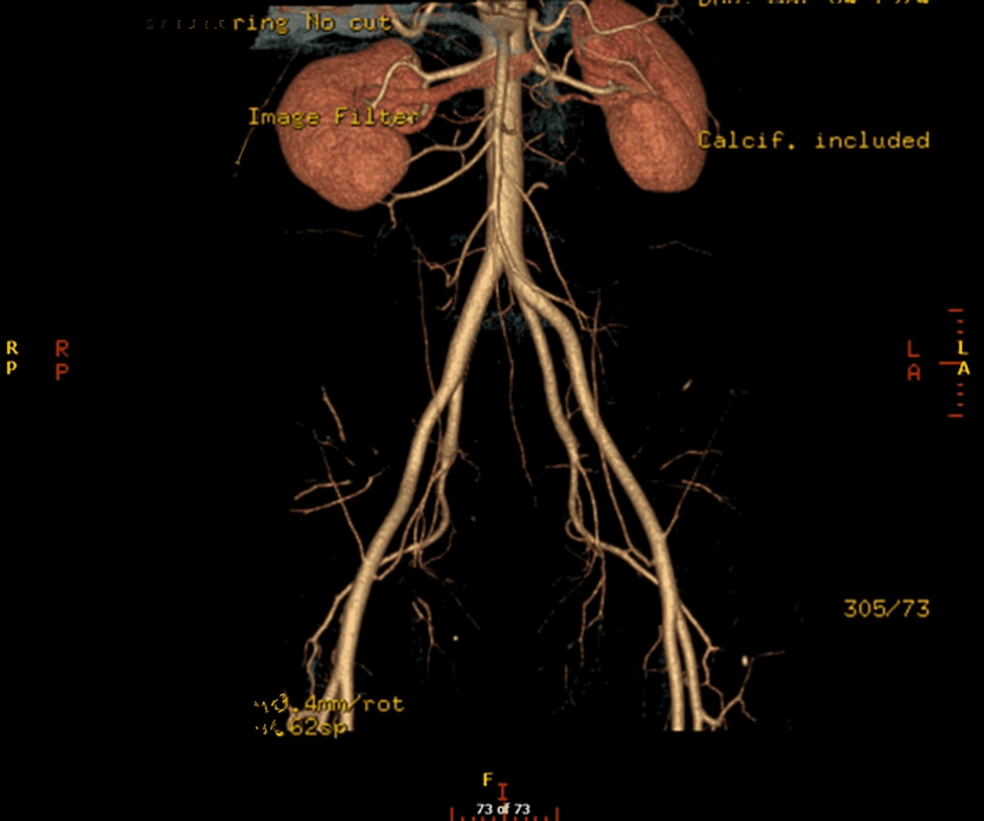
Three-dimensional reconstruction of an abdominal CT with an angiogram showing the kidney’s arterial structure, demonstrating the right and left kidneys with a single artery. The length of the right renal artery from the aorta to the bifurcation is 2.6 cm. The length of the left extrahilar renal artery from the aorta to the bifurcation is 1.8 cm CT: computed tomography
